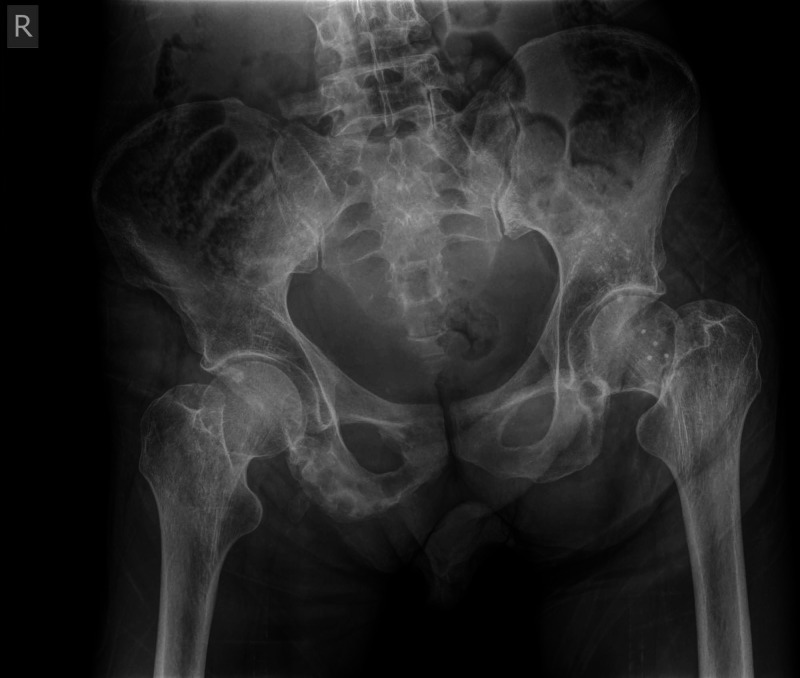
Preoperative anteroposterior pelvis X-ray demonstrating the subcapital fracture of the left hip
